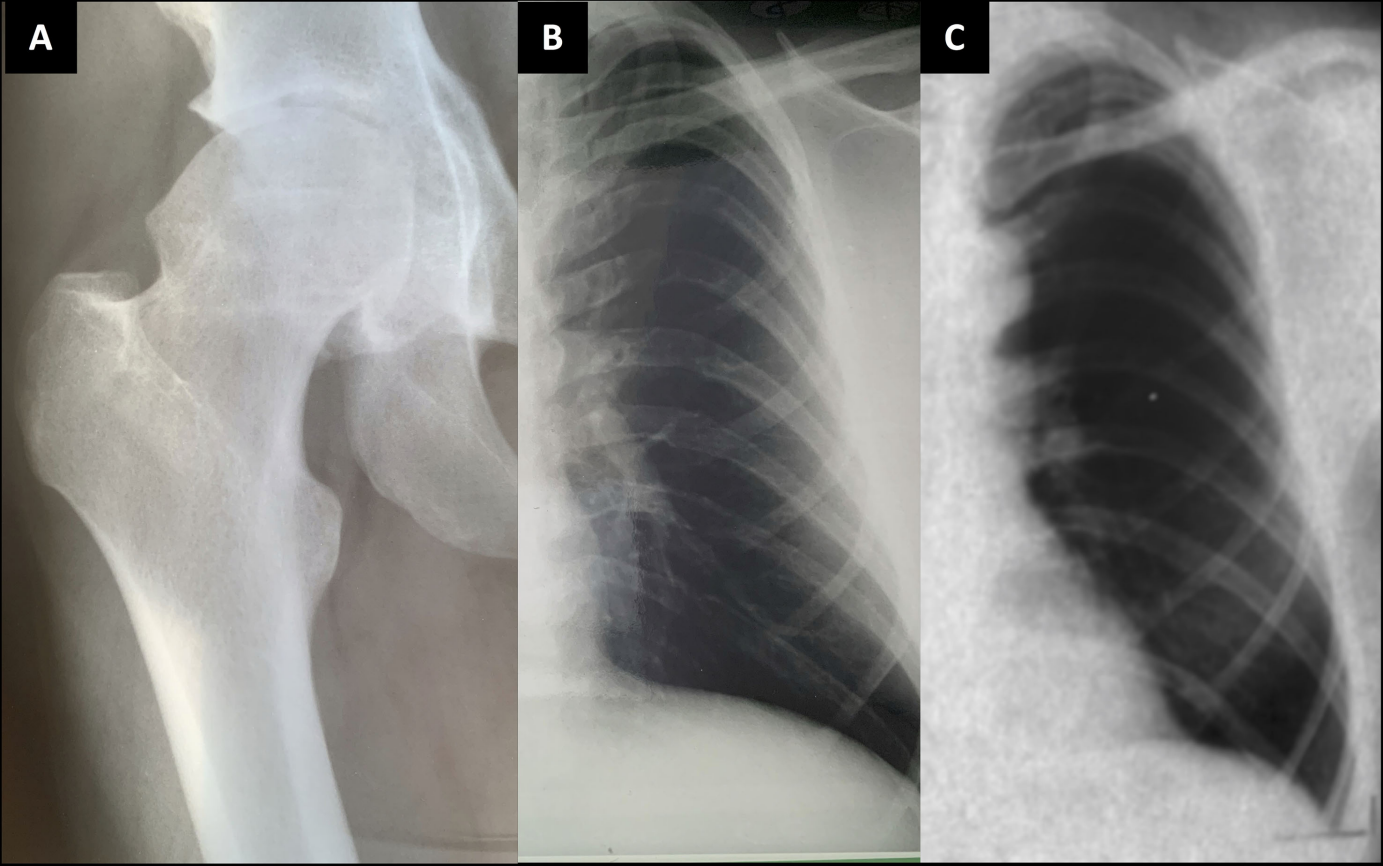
Plain radiographs of the index patient’s father and paternal grandfather. Index patient’s father (II-1) at age 24 (A, B); and the paternal grandfather (I-1) at age 41 (C). Images show slender diaphyses in ribs and degenerative changes on articular surfaces in hip joint with acetabular osteophytes and sclerosis and uneven shape in femoral head.
